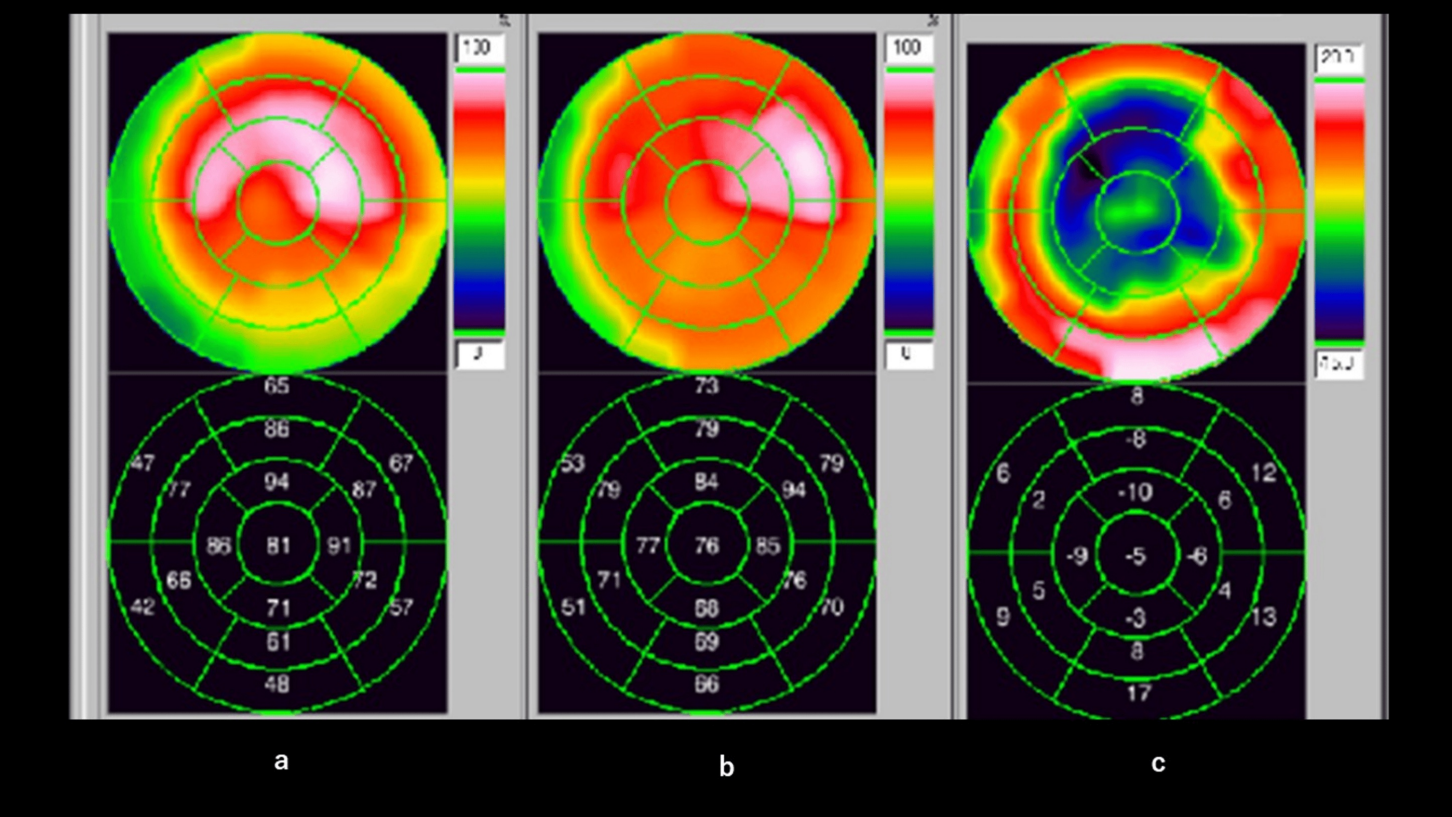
Cardiac scintigraphy with Tl-BMIPP Myocardial scintigraphy did not show a pattern of reduced blood flow along the coronary artery territory, as seen with vasospastic angina. a: thallium (Tl) scintigraphy, b: iodine-123 β-methyl-p-iodophenyl-pentadecanoic acid (BMIPP) scintigraphy, c: images of a and b
